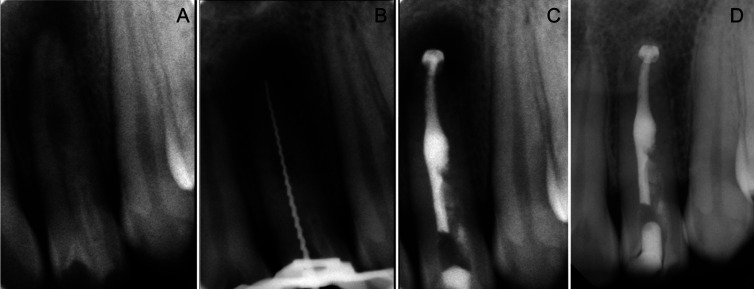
A. Initial X-ray. B. Working length X-ray determination; C. MTA apical plug and gutta-percha backfill; D. Radiographic follow-up at 8 years.
